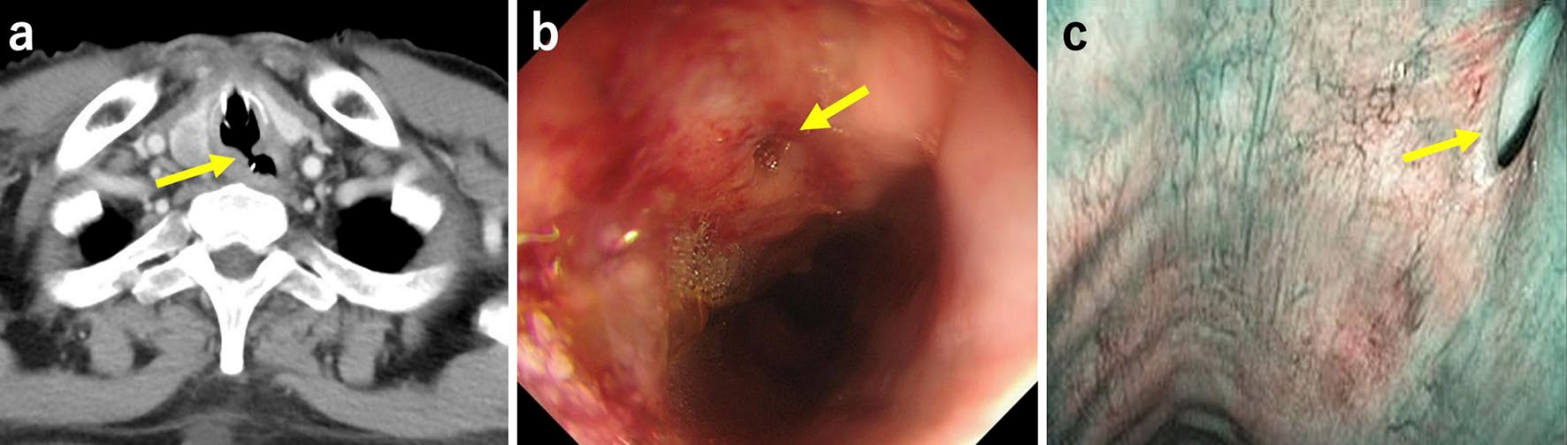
Contrast-enhanced CT (a), upper endoscopy (b), and narrow band imaging bronchoscopy (c) images after one course of DGS therapy. The primary tumor had shrunk, which caused formation of the cervical TEF (yellow arrows) (colour figure online)
